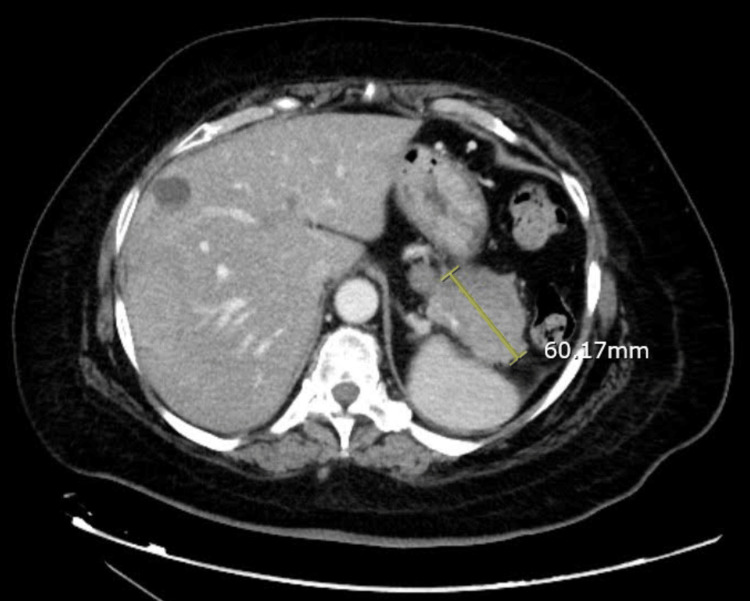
CT chest, abdomen, and pelvis transverse section displaying a 6.0 cm pancreatic tail mass with multiple liver metastases
